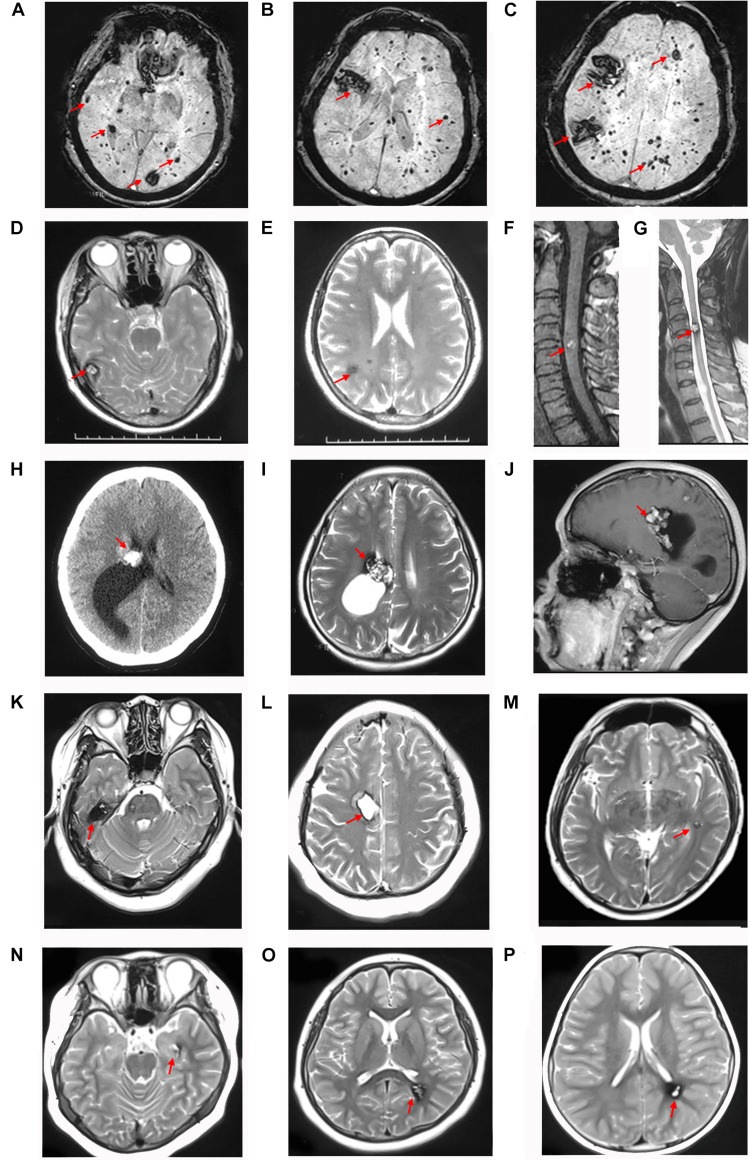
CCM lesions diagnosed through CT, T2-weighted MRI and SWI. (A–C) SWI of patient I-2 showed hundreds of lesions distributed across the cerebral hemisphere and cerebellum. T2-weighted MRI of patient II-4 showed multiple CCMs in the right temporal lobe (D), frontal and parietal lobes (E), and spinal cord (F,G). CT (H), T2-weighted (I), and contrast MRI (J) of patient II-5 showed lesions with a “popcorn” appearance located in the right lateral ventricle and frontal-parietal lobe with asymmetrical ventricles. (K,L) T2-weighted MRI of patient III-1 showed lesions surrounded by a dark rim of hemosiderin on the right frontal and temporal lobes. (M) T2-weighted MRI of patient III-2 showed a lesion located on the left temporal lobe. (N,O) T2-weighted MRI of patient IV-1 showed lesions on the left temporal lobe and triangle area of the lateral ventricle. (P) T2-weighted MRI of patient IV-2 showed a lesion located in the left triangle area of the lateral ventricle. The red arrows show the CCM lesions.
